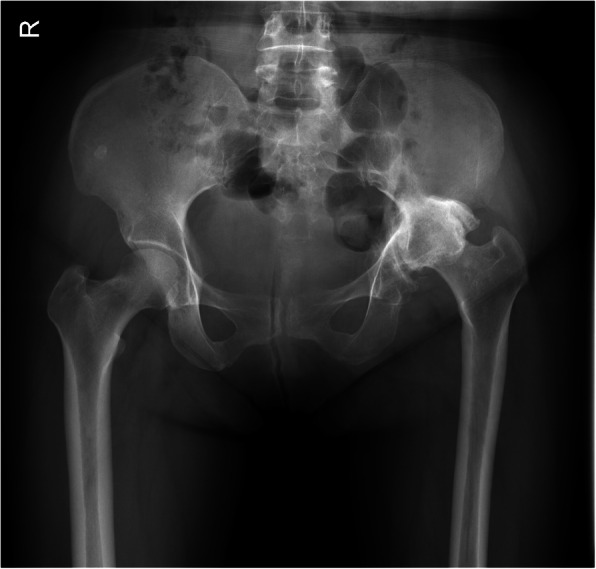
A 52-year-old female patient had been referred because of left hip pain for the past year. She had reported a previous “fall from a height” 3 years ago, which caused an “acetabular fracture”. The patient received conservative treatment. The radiograph showed “osteoarthritis” of her left hip joint, a condition that is apt to be misdiagnosed
